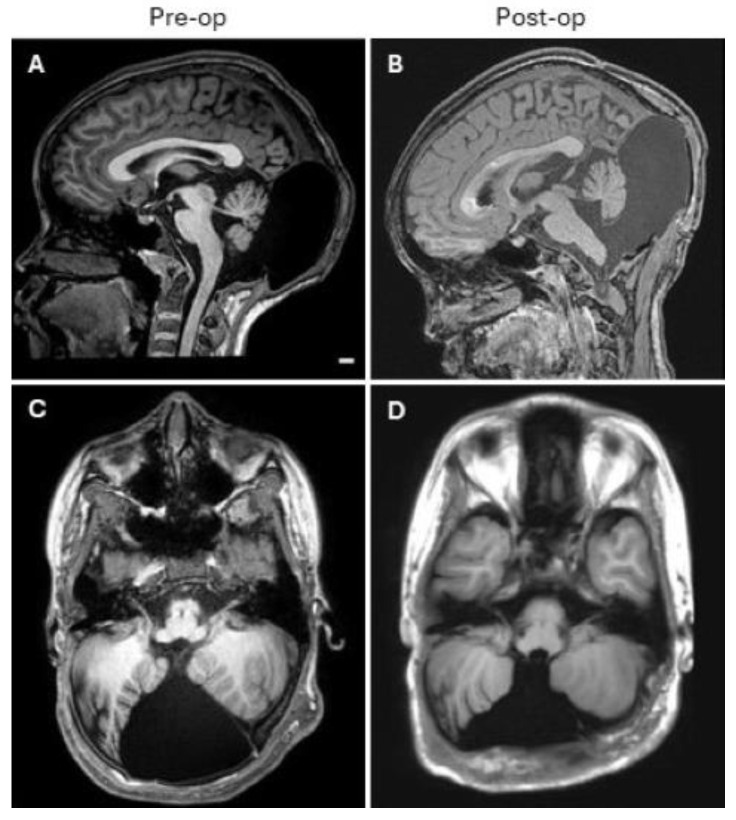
Sagittal and axial T1 MR imaging at 15 years of age. (A,C) Before the second surgery, it shows a large arachnoid cyst of the cisterna magna protruding beyond the margins of the defect inferiorly. (B,D) One month later, it shows a decreased external herniation of the cyst and an increased soft tissue between the margin of the cyst cavity and the skin.
